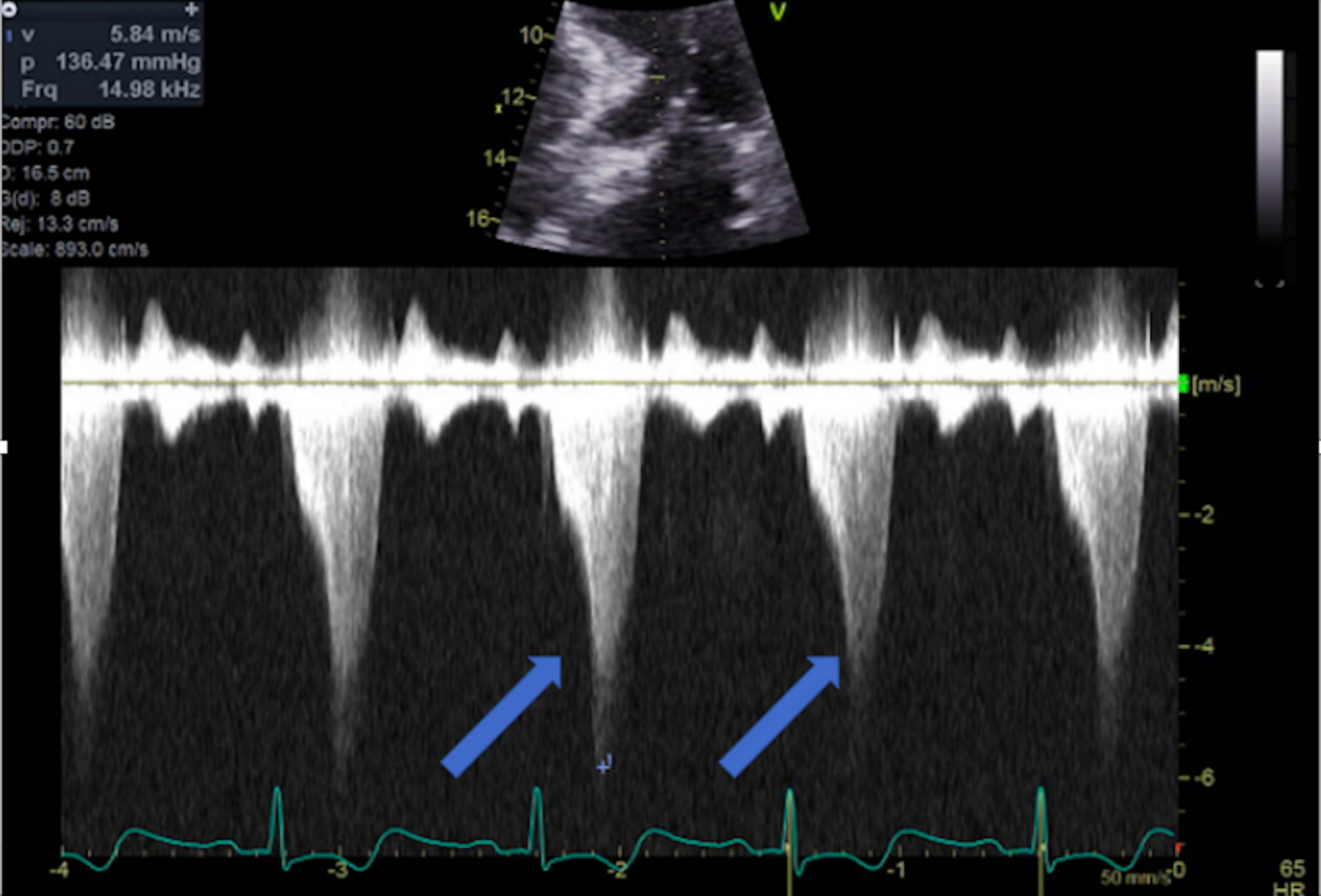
CW Doppler across LVOT at rest showing an increased pressure gradient CW Doppler: continuous Doppler; LVOT: left ventricular outflow tract
